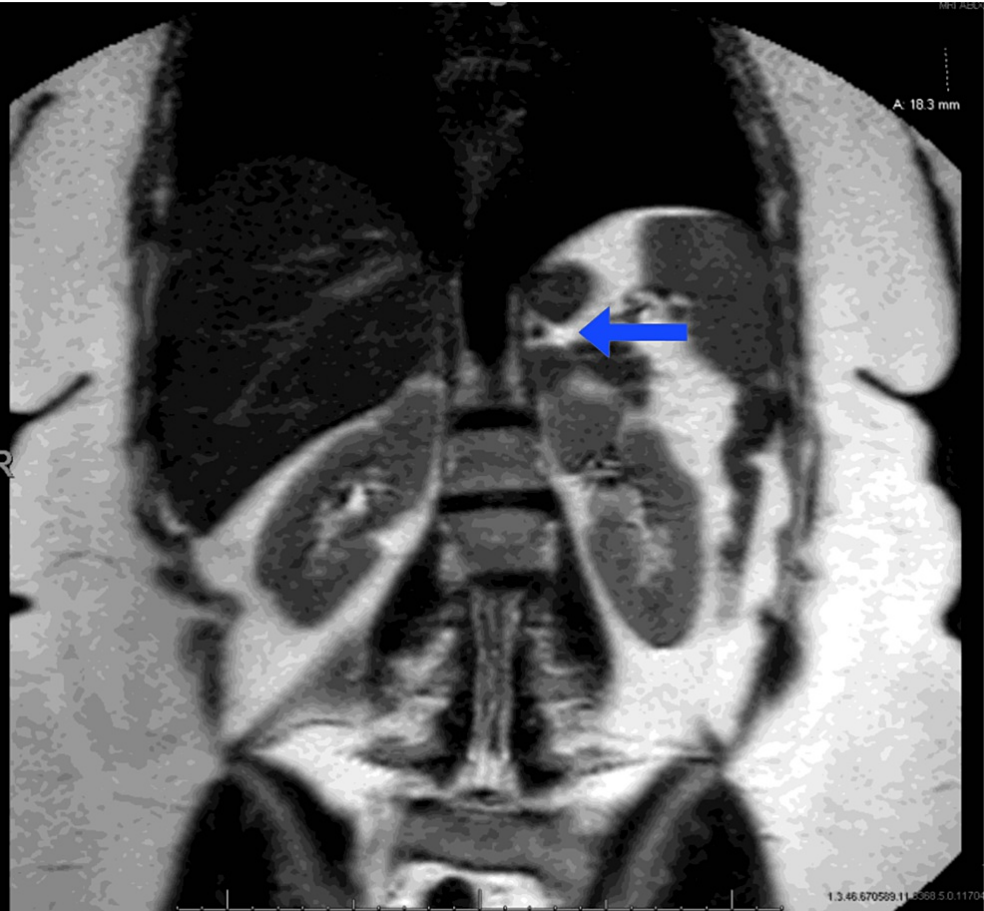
Abdominal magnetic resonance imaging (MRI) scan. Arrow points to a clearly visible 3 cm pheochromocytoma in the left retroperitoneal flank region of a 45-year-old man who presented with a history of episodic headaches, palpitations, and sweating for the past six months.
